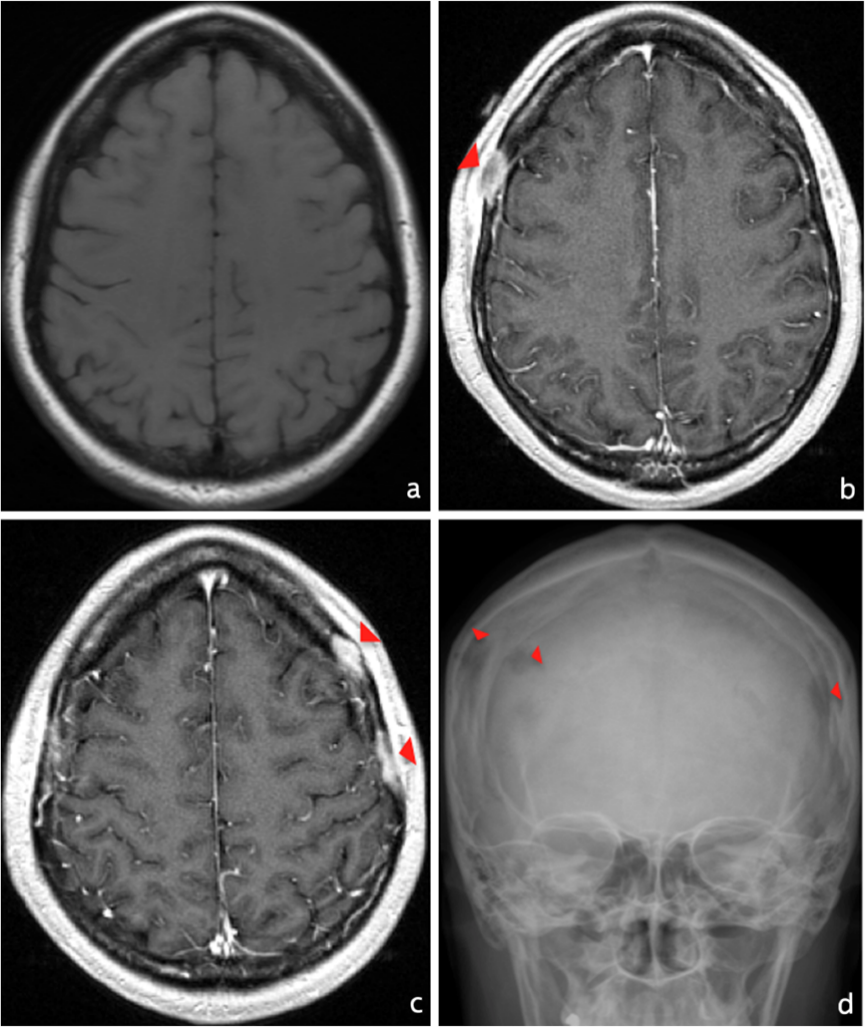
a- Normal pre-allogeneic HSCT MRI of brain.b and c- Post contrast 3D spoiled gradient echo showing numerous thickened contrast enhancing lesions measuring up to 1.6 × 0.8 cm in the left frontal, 2.0 × 0.9 cm in the right frontal and 2.7 × 0.5 cm in the left parietal region, within the calvarium. d- X-ray of skull, frontal view showing lytic lesions in the frontal and parietal bones.
